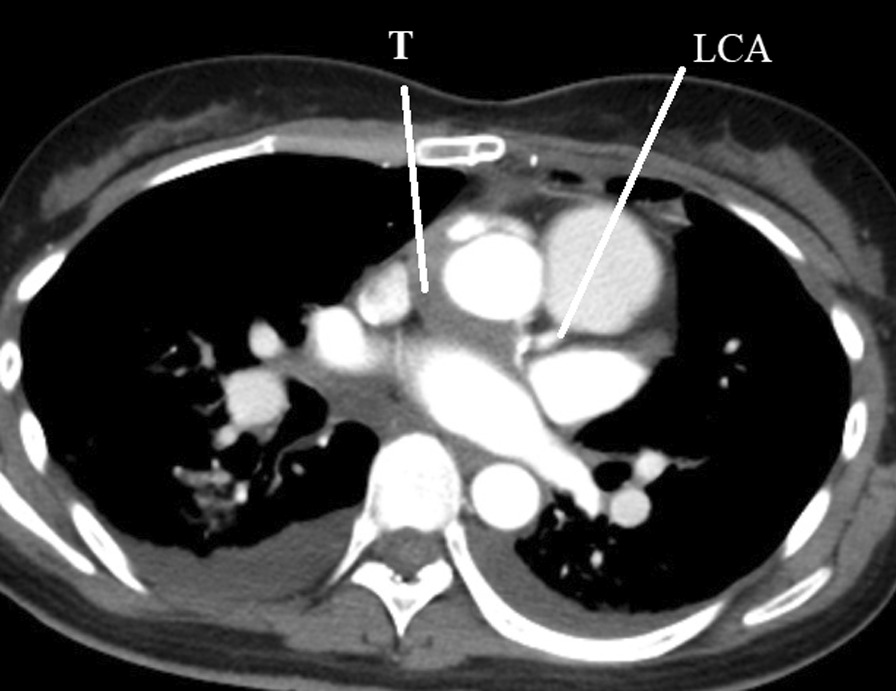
Contrast-enhanced computed tomography revealed that the interposing artificial graft to the left coronary artery was occluded by a thrombus. T, thrombosis in the interposing graft; LCA, left coronary artery
